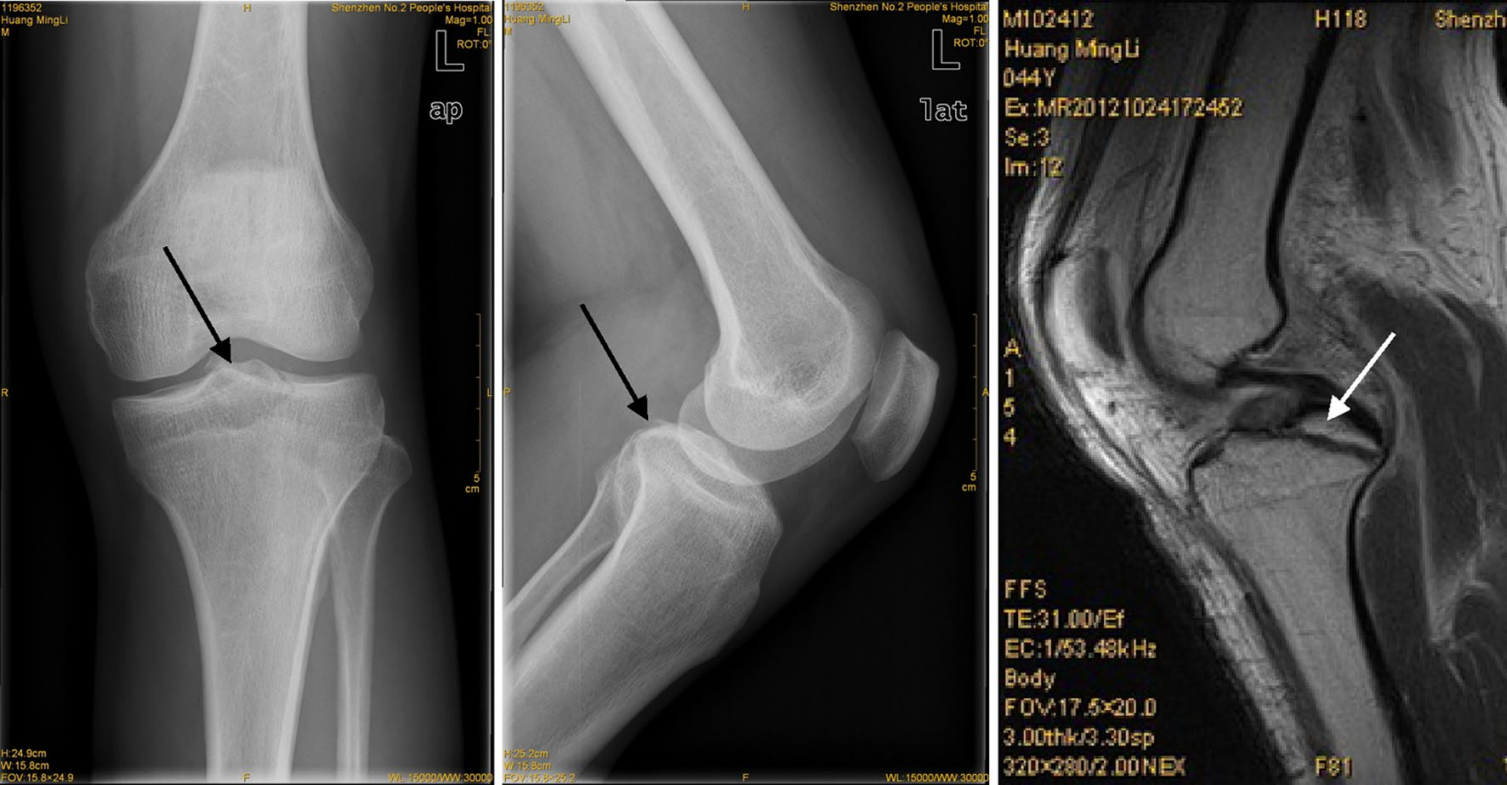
Preoperative X-ray and MRI examinations. Preoperative X-ray and MRI examinations indicate the avulsion fracture at the distal insertion of the posterior cruciate ligament (represented by the *arrow*)
